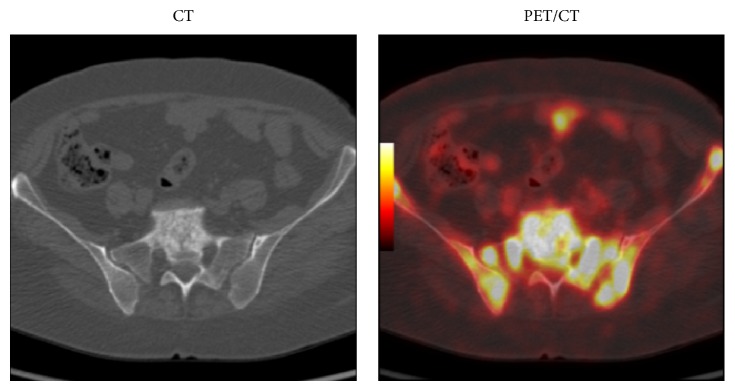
Breast cancer patient (35 years old) evaluated by pretreatment F18-FDG-PET/CT showing multiple bone marrow metastatic lesions in pelvic bones with concomitant early development of osteoblastic metastatic bone lesion in S1 vertebral body.
